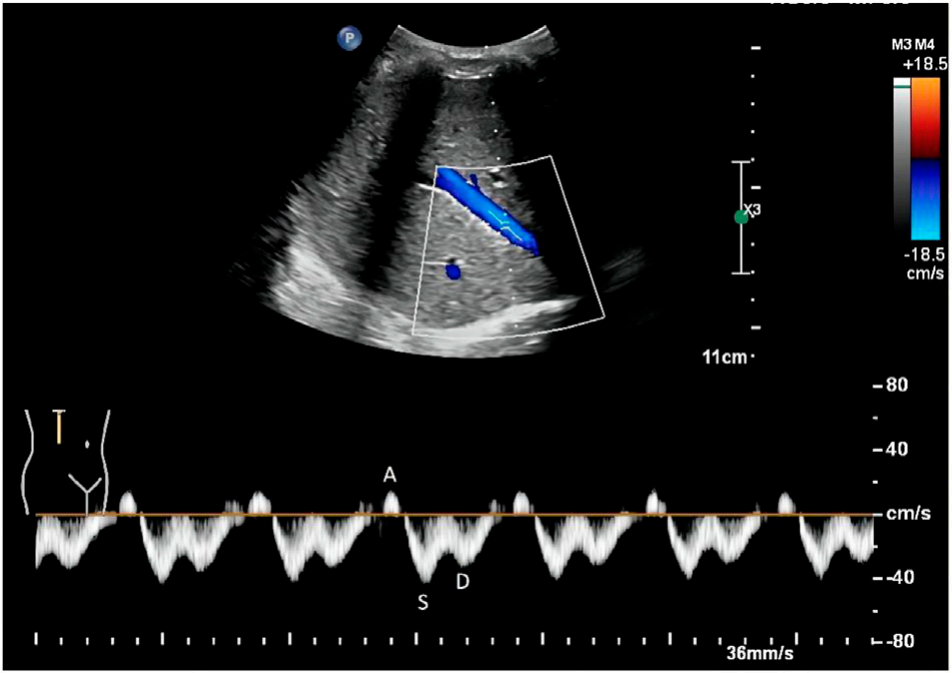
Normal hepatic vein flow pattern detected by Doppler. The hepatic vein has a triphasic waveform, which consists of an A wave above the baseline (representing atrial systole), and two waveforms below the baseline (S and D, representing venous return during ventricular systole and diastole, respectively).
